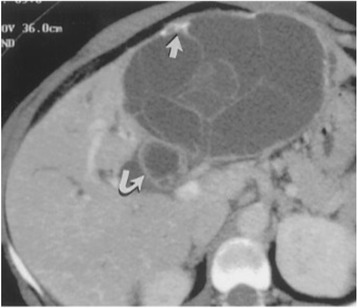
Computed tomography scan shows a multilocular cyst with septations and mural calcifications (*straight arrow*) with biliary duct dilatation and extension of the cyst into the left hepatic and common bile ducts (*curved arrow*) [9]
